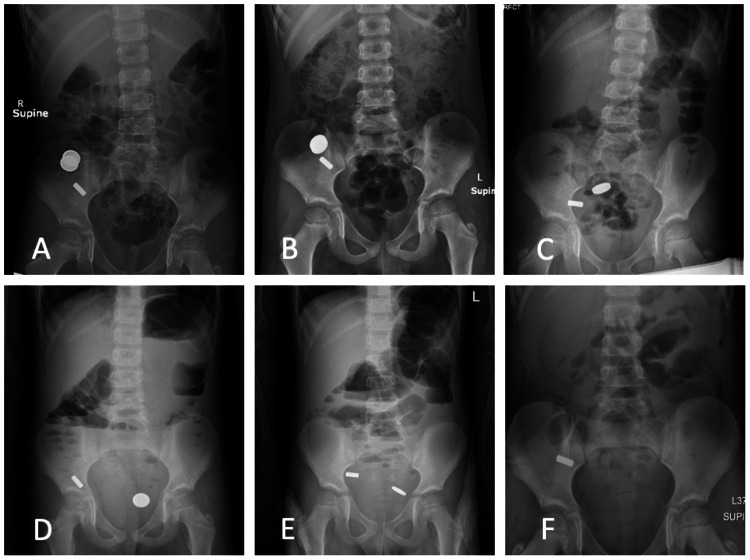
Progression through the gastrointestinal tract of ingested pennies (two) and inability to pass magnets (four) viewed via serial abdominal X-rays
